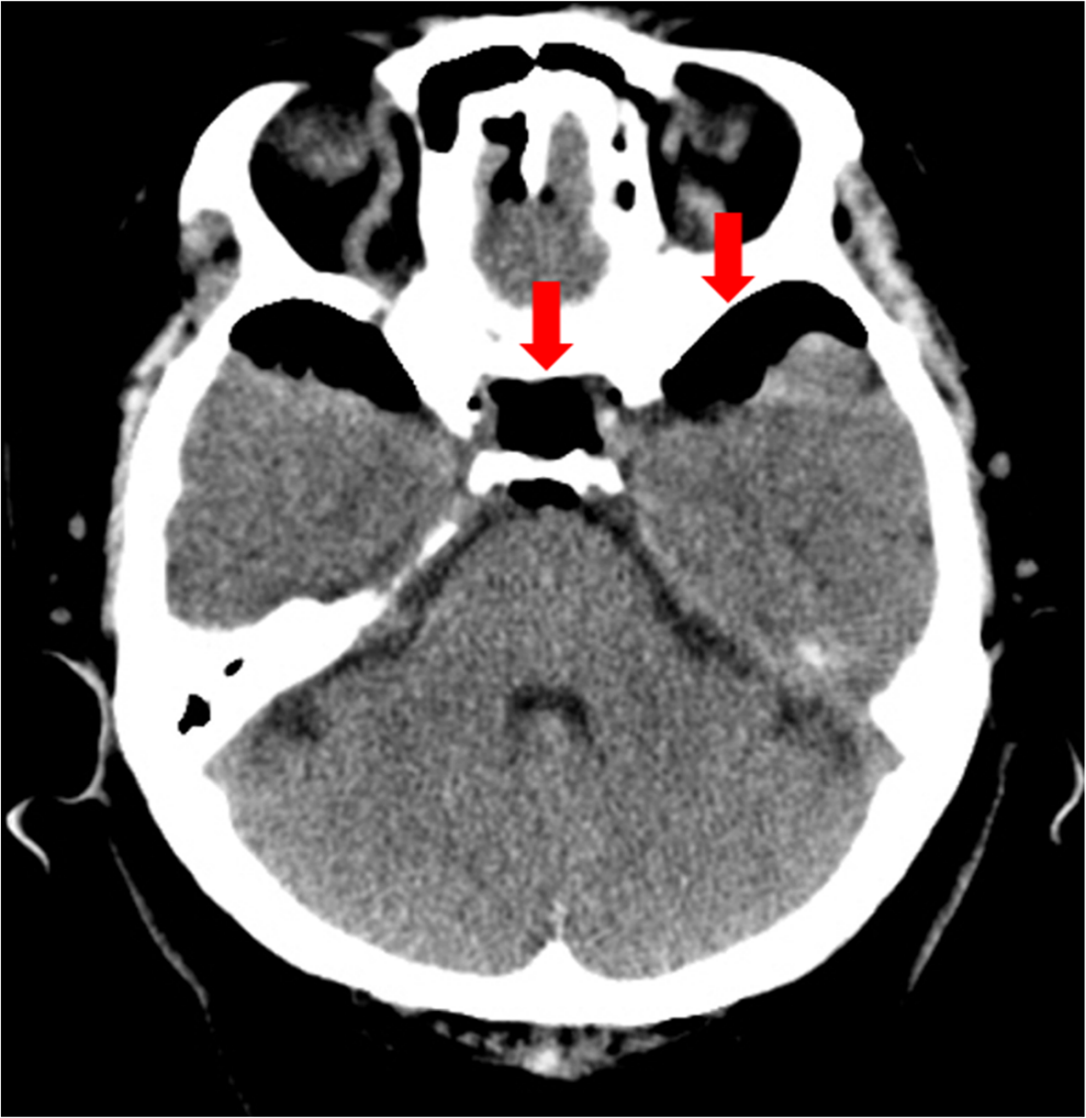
Brain computed tomographic scan demonstrating extensive anoxic brain damage and extensive pneumocephalus (red arrow), bilateral middle and lower frontal convexity and Sylvian fissures
